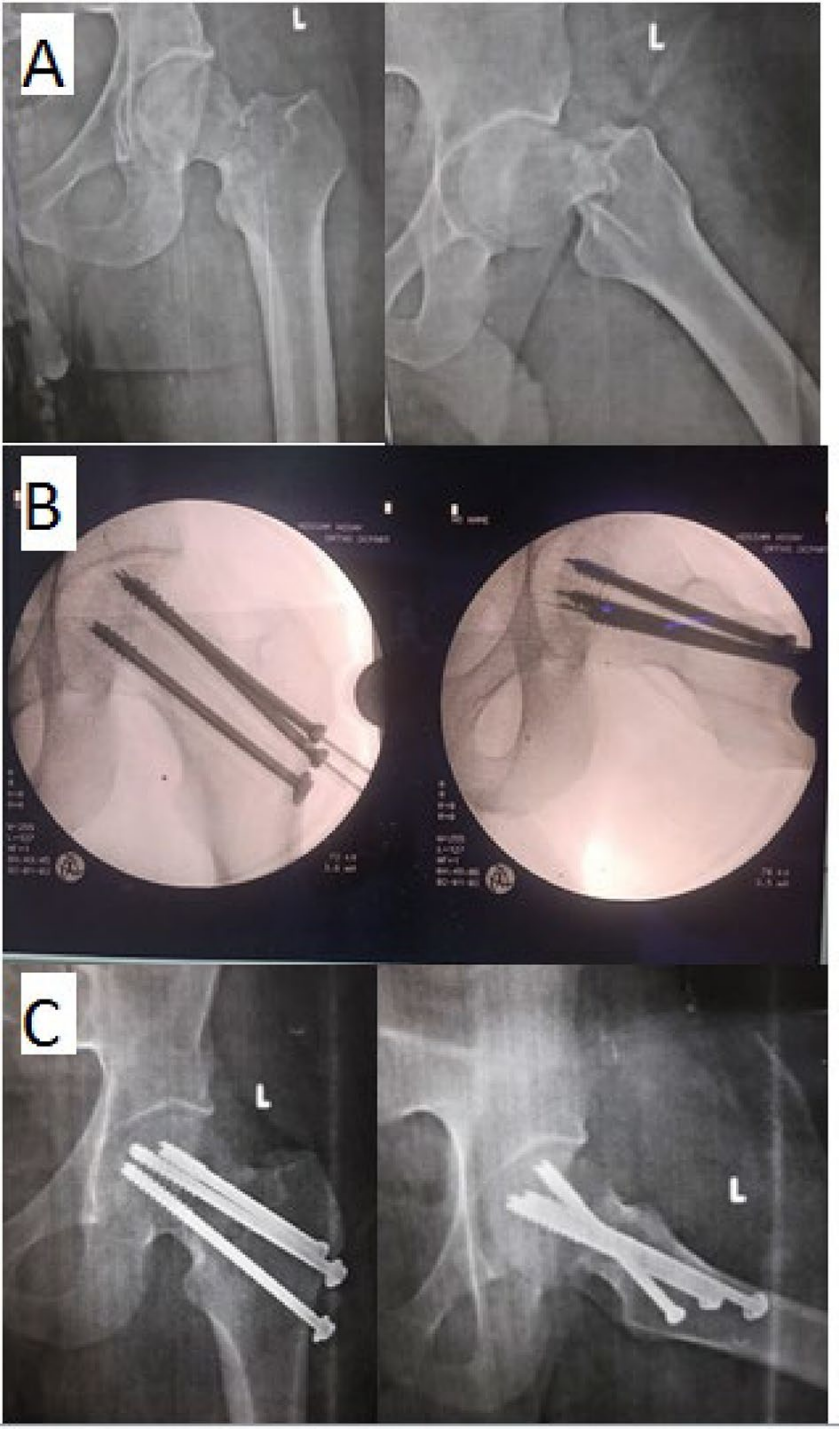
Thirty eight years old male patient with femoral neck fracture was resulting from falling from height and treated by MCCS. A Pre-operative plain x-rays showing transcervical femoral neck fracture of Garden type IV and Pauwels type III. B Intra-operative fluoroscopy showing confirmation of reduction and adequate fixation. C Immediate post-operative radiographs showing satisfactory reduction and fixation
